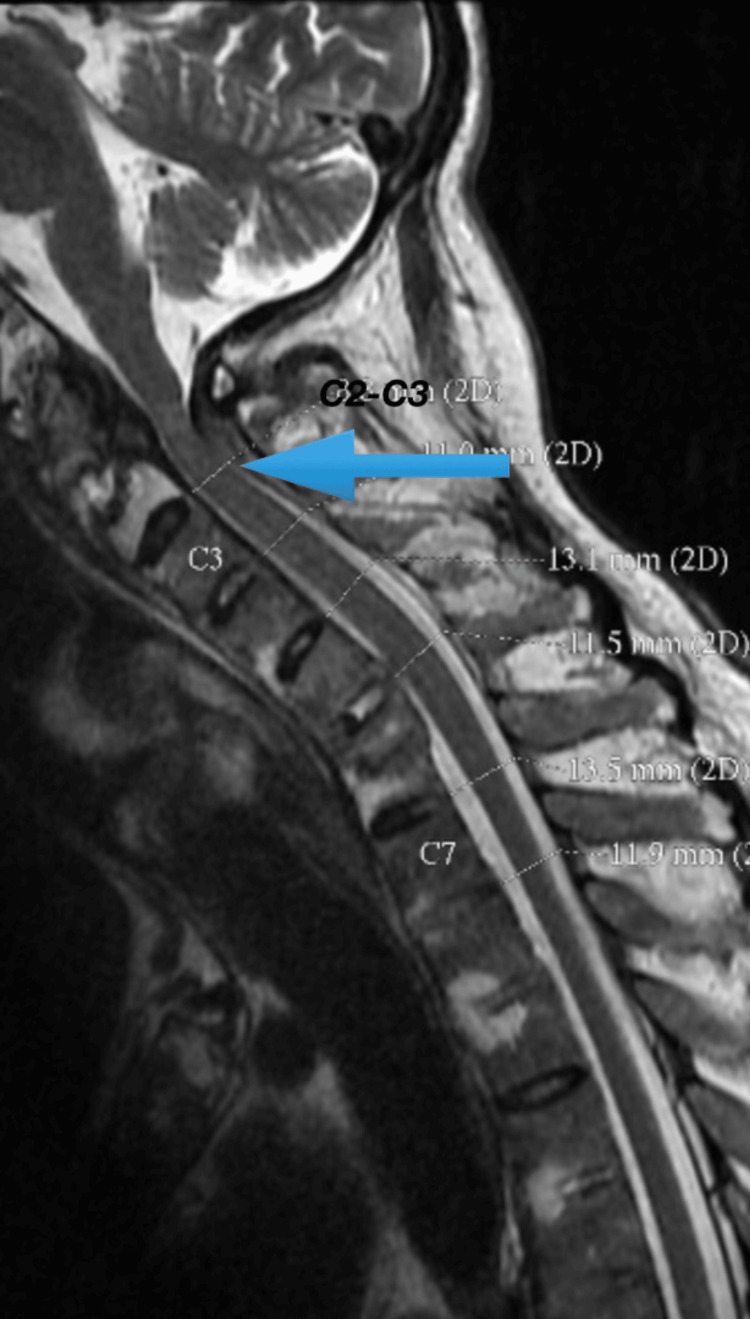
Sagittal T2-weighted MRI of the cervical spine depicting syndesmophytes and ossification of the anterior longitudinal ligament with significant spinal canal narrowing at C2-C3 (8.8 mm), raising concern for cervical canal stenosis in a patient with ankylosing spondylitis The blue arrow shows the changes of ankylosing spondylitis which is causing cord compression at the level. MRI: magnetic resonance imaging; C2: second cervical vertebra; C3: third cervical vertebra
